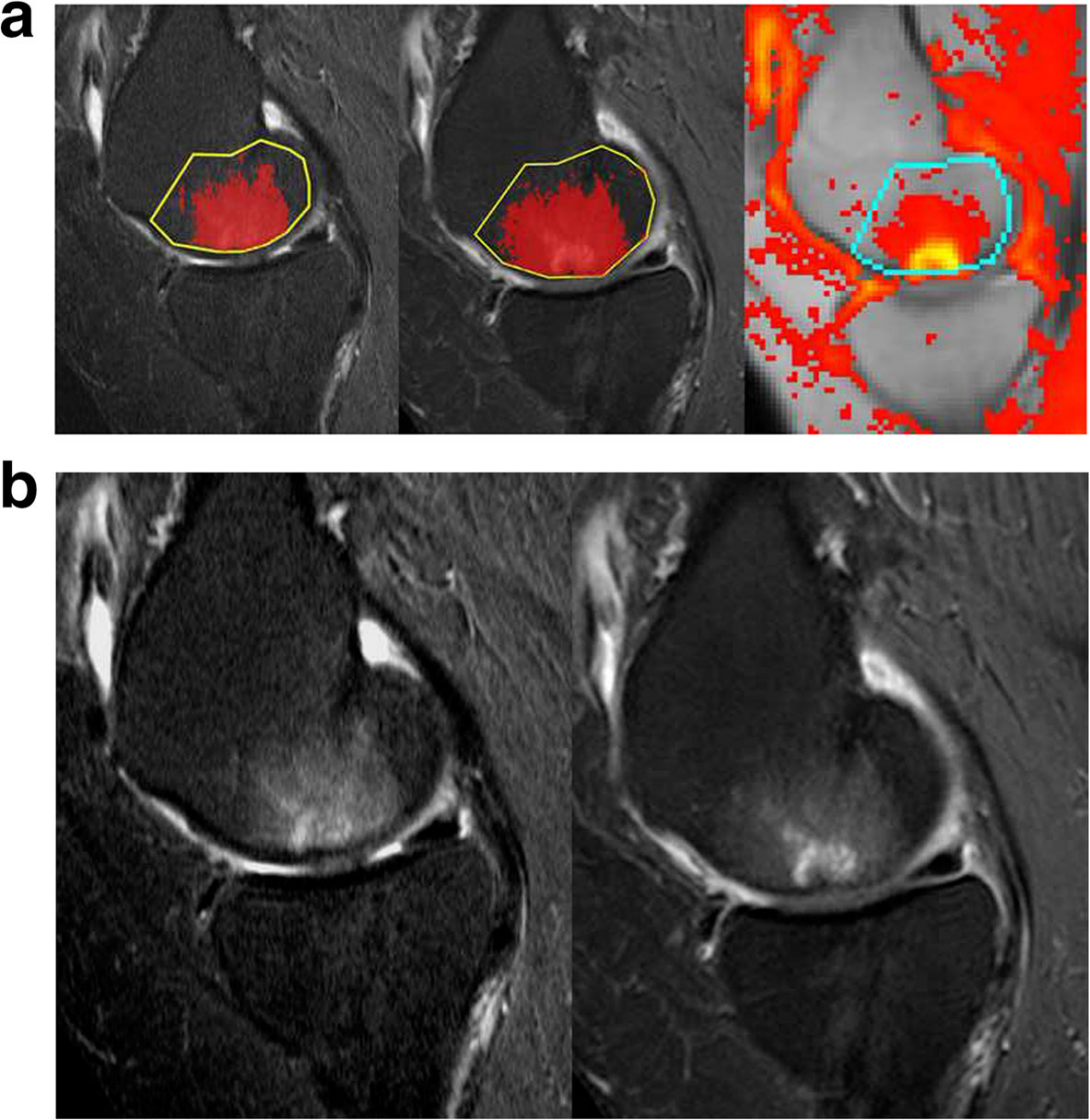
a Sagittal STIR (*left*), post-contrast T1 FS (T1 CE FS) (*middle*) and dynamic contrast enhanced MR images (DCE-MRI) (*right*) in one patient through the medial joint compartment with region of interests (ROIs) drawn in the weight-bearing femoral condyles. Computer assisted segmentation of BML on the STIR and T1 CE FS images is shown; the marked pixels represent areas above the threshold signal intensity, i.e., STIR-BML and CEA-BML, respectively. An area with rapid and steep enhancement (right image) is seen in the femoral condyle, *yellow* color. Pixels exhibiting a pathologic enhancement pattern within the ROI are visualized and segmented digitally. b Corresponding STIR (left) and T1 CE FS images (*right*) without ROI or pixel segmentation. (BML=bone marrow lesion, CEA-BML=contrast enhancing areas of bone marrow lesion)
